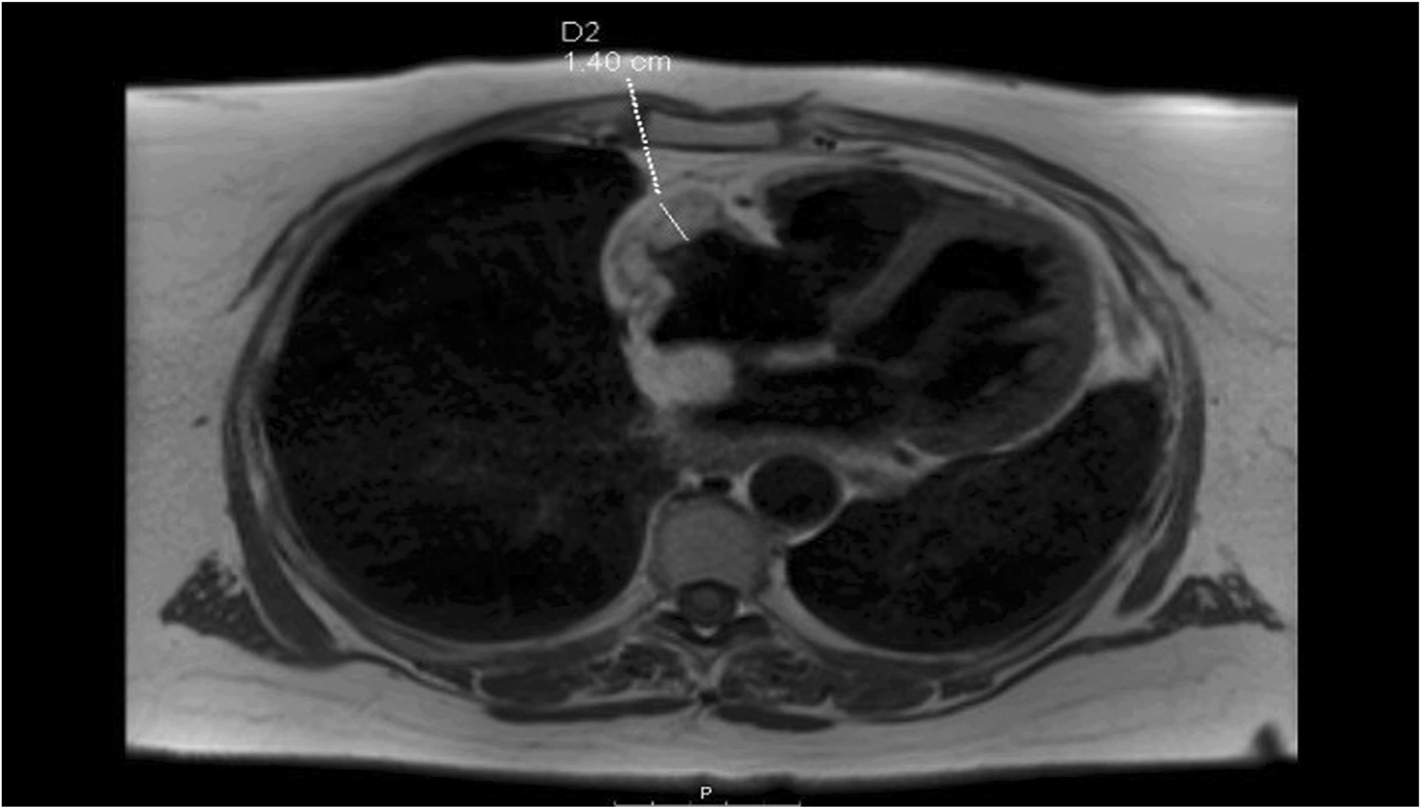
Transverse T1 weighted Cardiac MRI showing right atrial mass
